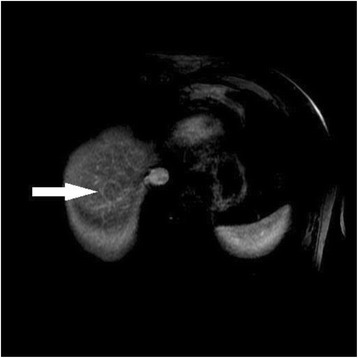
67 year old male with alchohol liver disease and cirrhosis. Venous phase MRI with gadolinium demonstrates an HCC nodule at the dome of the liver with capsular enhancement
